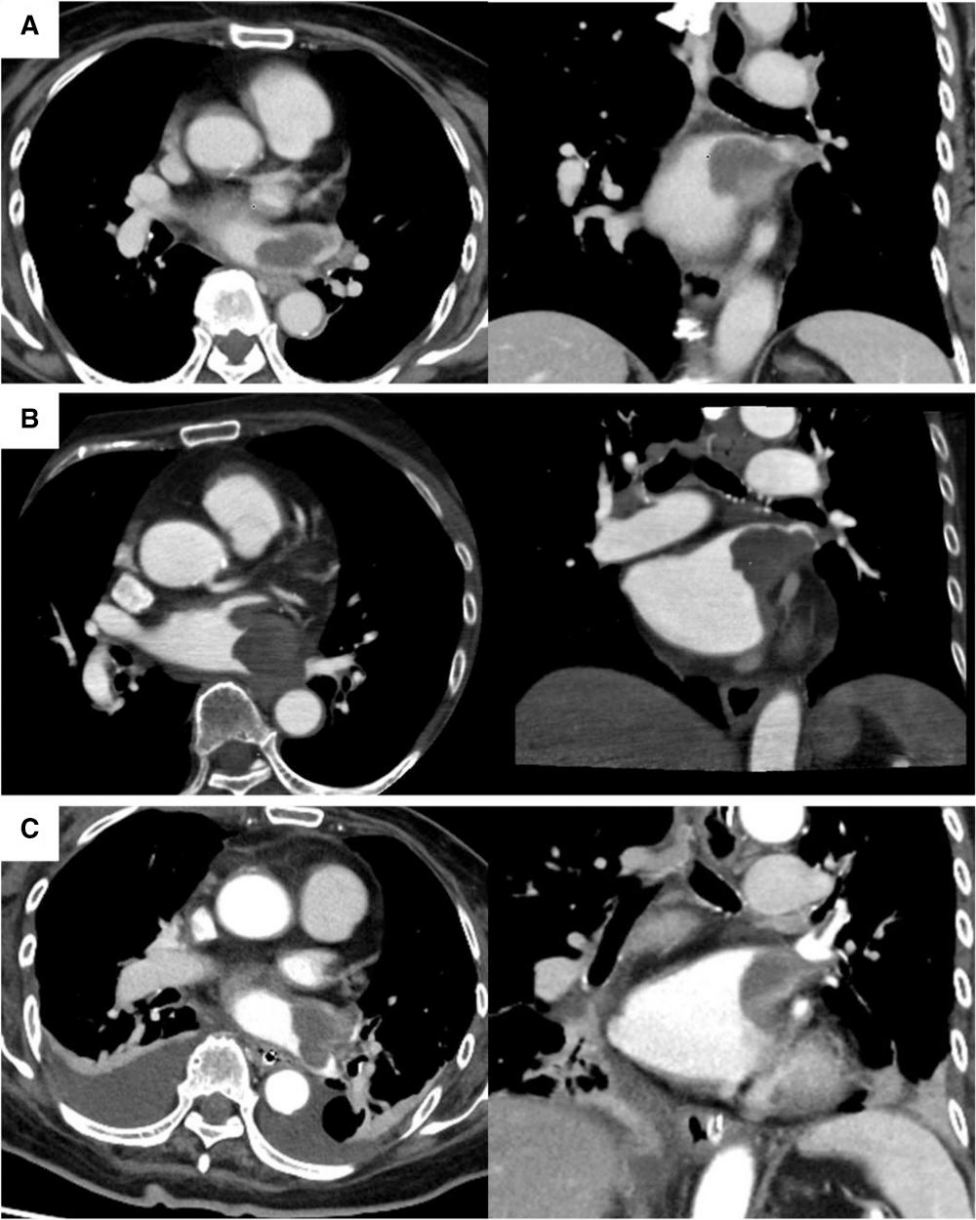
Contrast-enhanced computed tomography. (*A*) On Day 4, a contrast defect is visible in the left upper and lower pulmonary veins, with no primary tumours or lymphadenopathy detected elsewhere. (*B*) On Day 35, a broad-based exophytic mass obstructing the left pulmonary vein is visible, which appears larger than that in the previous imaging. (*C*) On Day 65, contrast-enhanced computed tomography shows tumour regrowth in the resected area, indicating local recurrence.
